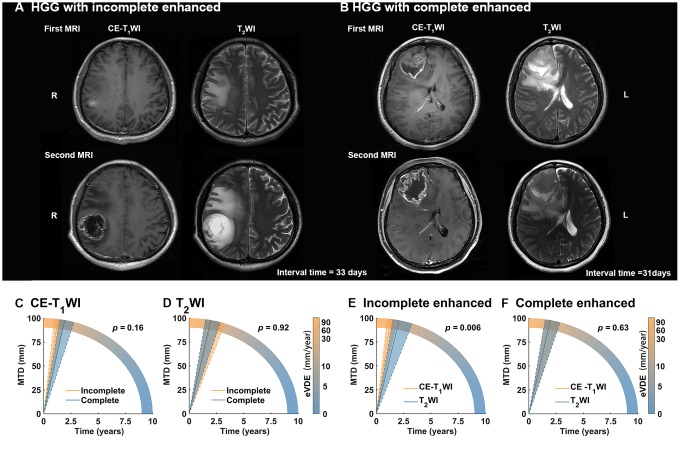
Tumor growth rate in different contrast enhancement (CE) type. (A, B) The longitudinal contrast-enhanced T_1_-weighted image (CE-T_1_WI) and T_2_WI MR-images with incomplete enhanced and complete enhanced high-grade gliomas (HGGs), respectively. (C, D) For HGGs with two or more CE- T_1_WI MR images (n = 54), equivalent velocity of diameter expansions (eVDEs) in different CE type based on T_2_WI (represented tumor entity) and CE-T_1_WI (represented tumor enhanced area) showed no significant difference (*p* > 0.05). (E, F) HGGs with incomplete enhanced showed significant faster eVDEs in tumor enhanced area than tumor entity (*p* = 0.006). However, HGGs complete enhanced showed no significant difference in eVDEs between tumor enhanced area and tumor entity (*p* = 0.63).
